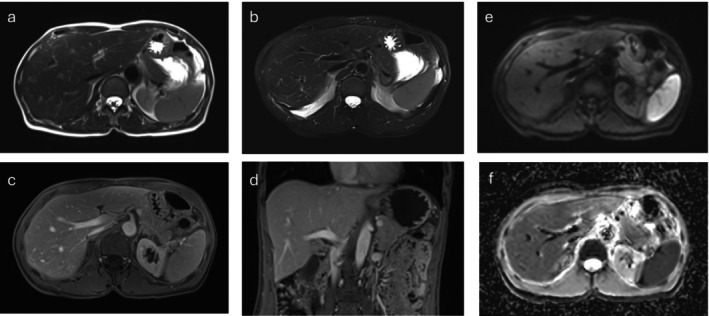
MRI of a 56‐year‐old female with suspected Morbus Crohn of Ileum. (a) Axial T2‐HASTE sequence, (b) axial DL‐HASTE sequence, (c) axial T1 sequence, (d) coronal T1 sequence, (e) diffusion map (Trace), and (f) diffusion map (ADC).
